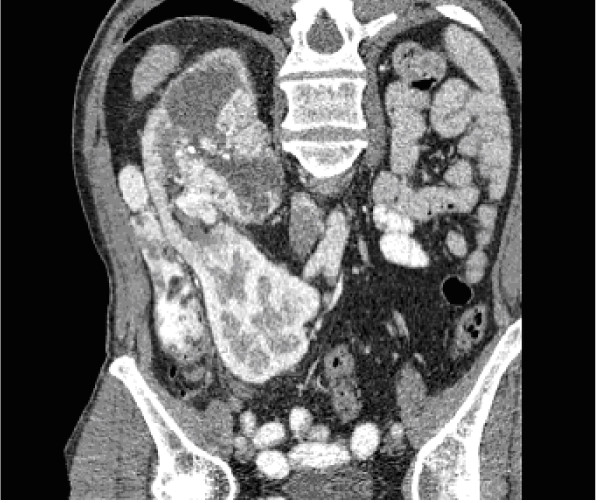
CT scan showing tumour in the upper moiety of the crossed fused renal ectopia.
